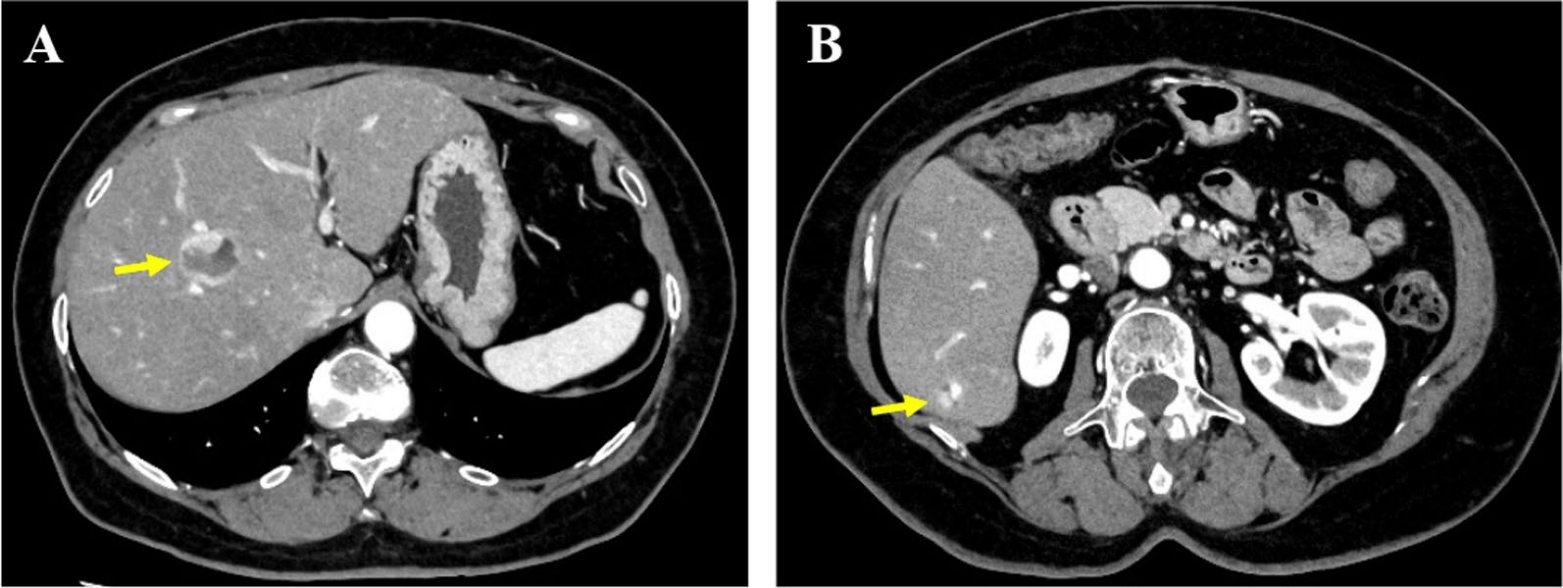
Contrast-enhanced computed tomography at the follow-up visit 2 years postoperatively. A A 26- × 24-mm mass in S8 (arrow) and B a 10- × 10-mm mass in S6 (arrow) were shown as hypervascular lesions in the early phase on contrast-enhanced computed tomography
